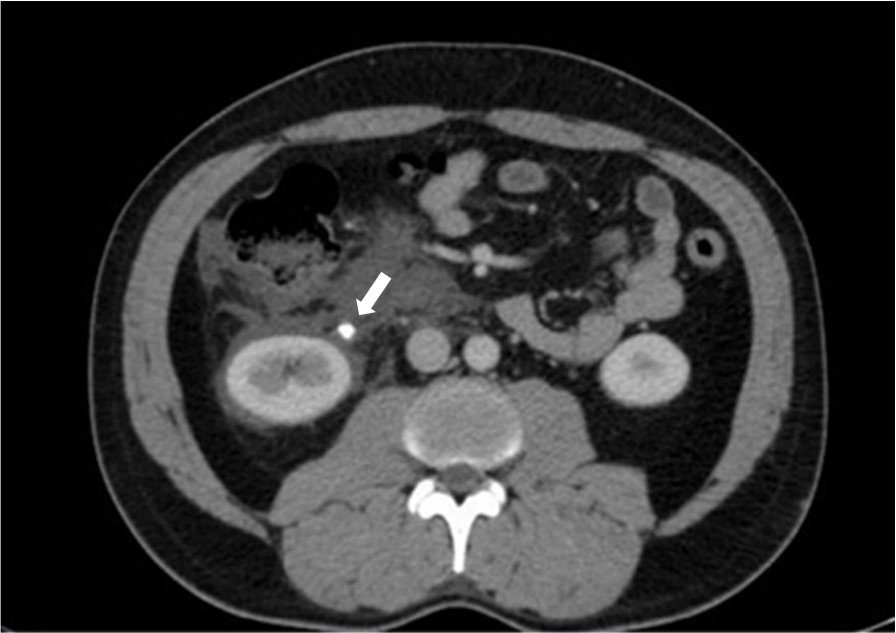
Abdominopelvic CT scan with contrast showing a small stone in the ruptured renal pelvis
